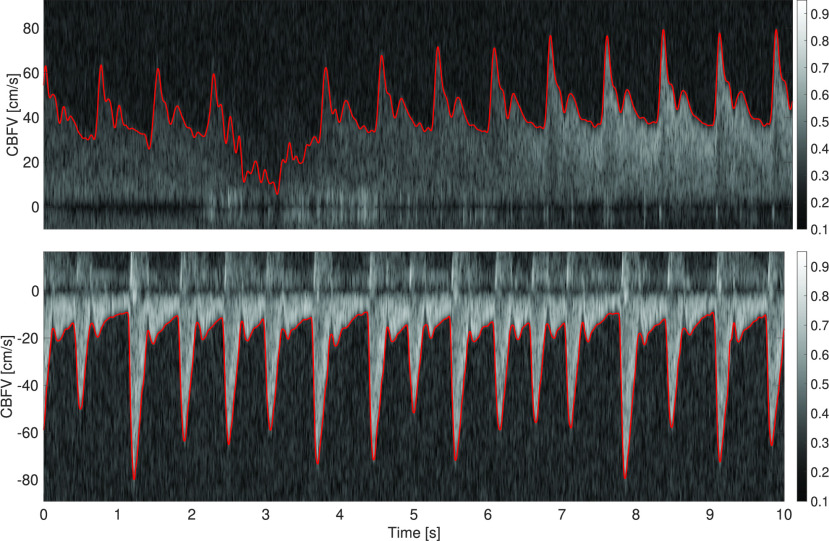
Top: Doppler spectrogram obtained by insonating the M1 segment of the middle cerebral artery. The frequency shift is converted to a velocity, such that the cerebral blood flow velocity (CBFV) is expressed in cm/s. The spectrogram shows varying levels of intensity and a signal loss between 2 s to 4 s. The algorithmically determined envelope is marked in red. Bottom: Doppler spectrogram and envelope obtained from the internal carotid artery.
